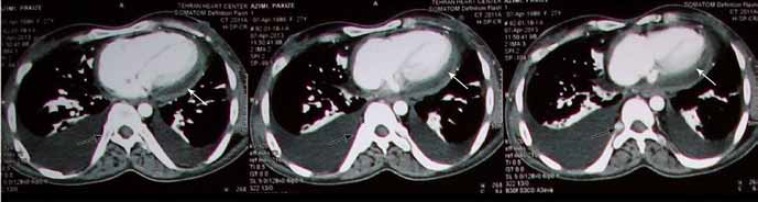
Chest CT scan shows increased pericardial thickness (white arrows) with an abnormal thorax and scoliosis of the vertebral column (black arrows).
